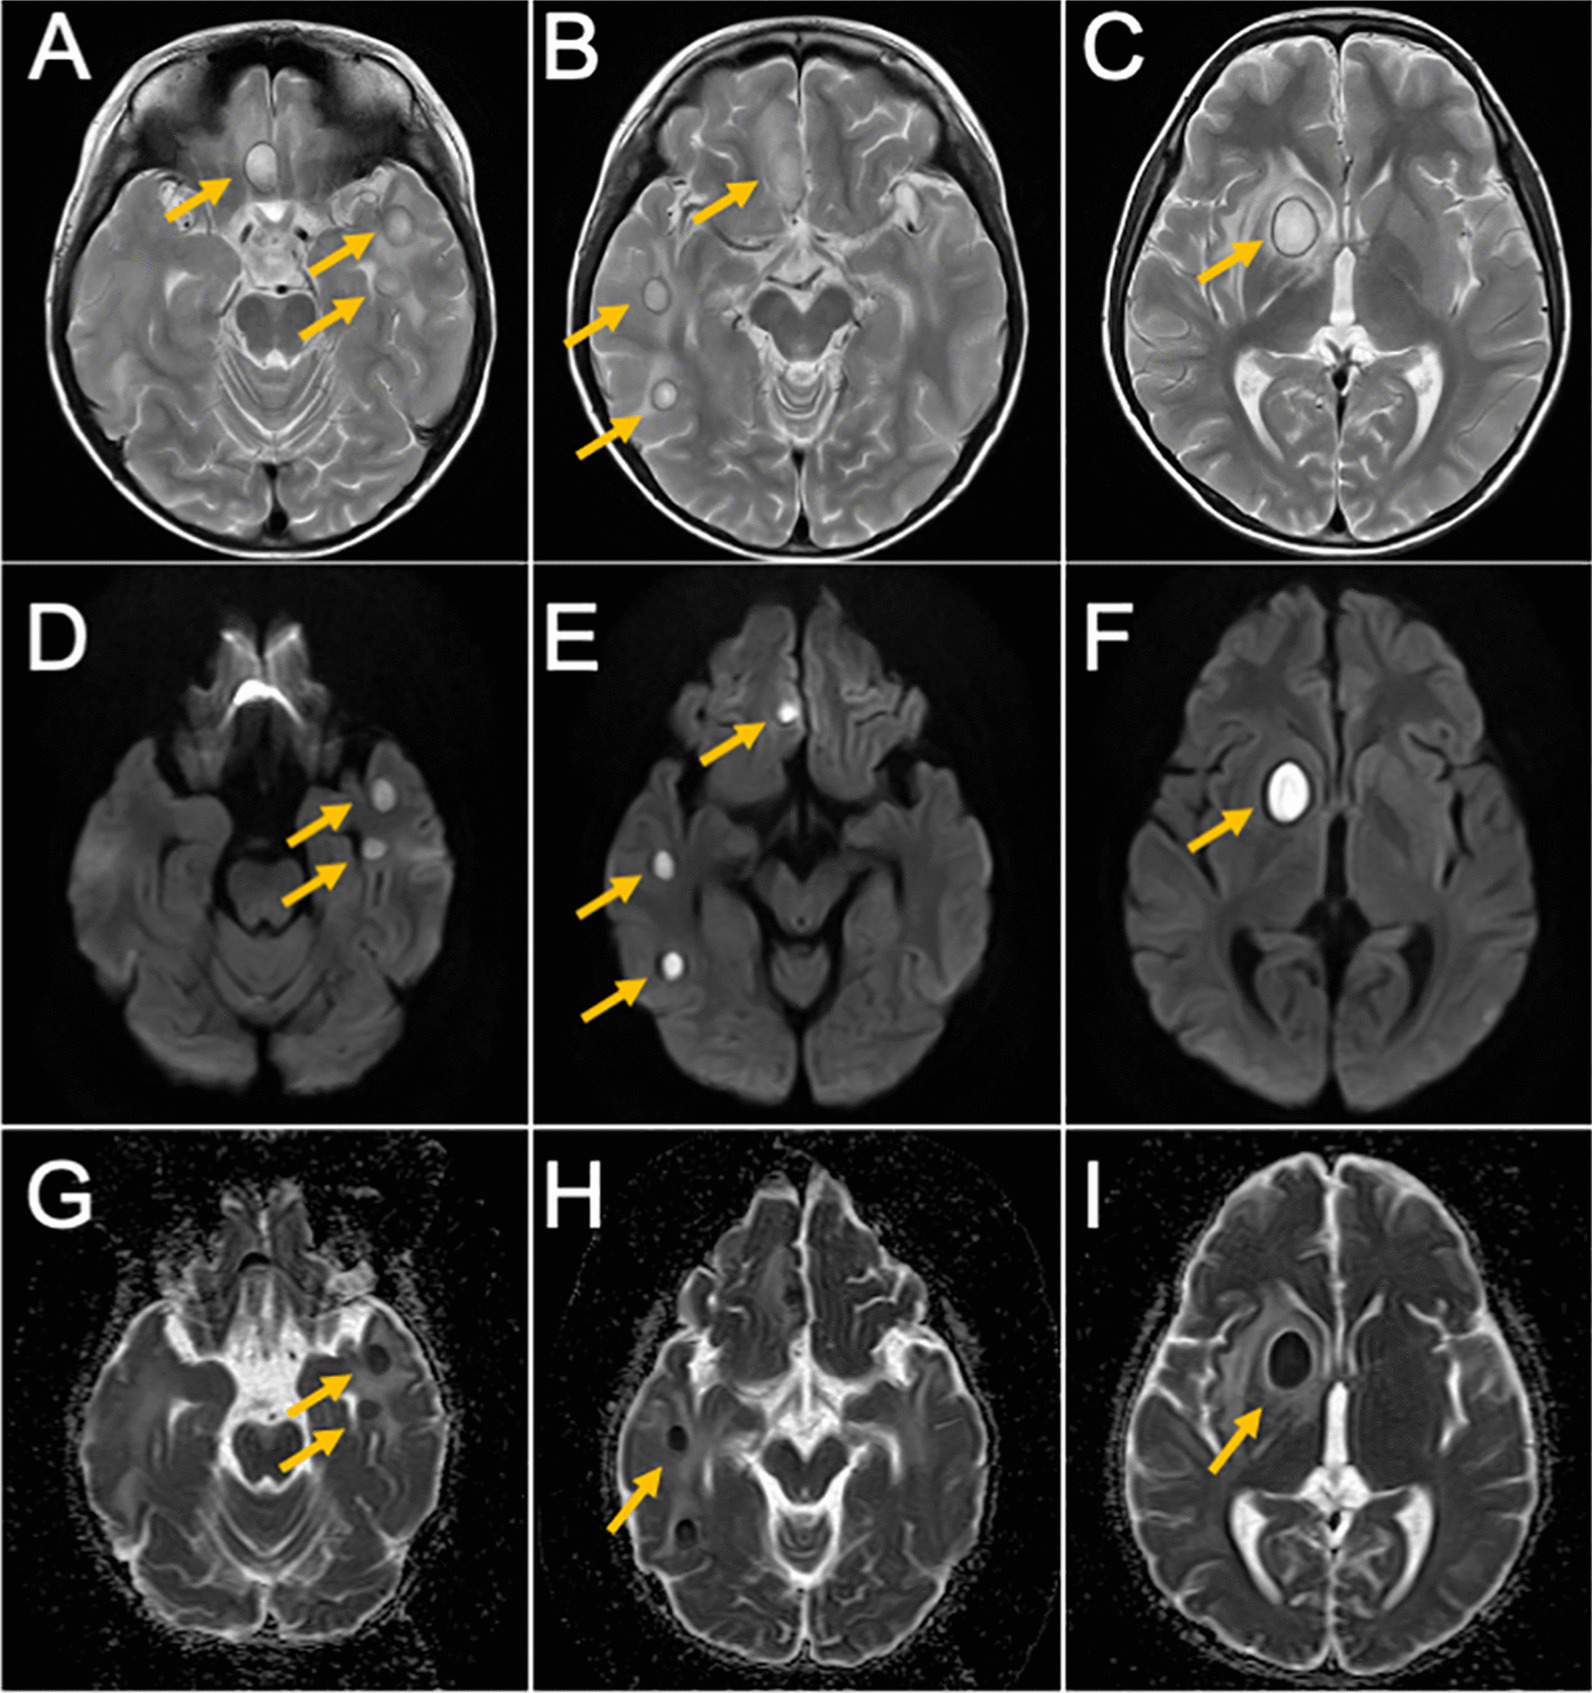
MRI obtained on September 26, 2020. T2-weighted image (A, B, and C), diffusion-weighted image (D, E, and F), ADC map (G, H, and I). Yellow arrows indicate brain abscesses. There were multiple nodular lesions showing ring-like hyperintensity in T2-weighted images and internal hyperintensity and decreased ADC in diffusion-weighted images
